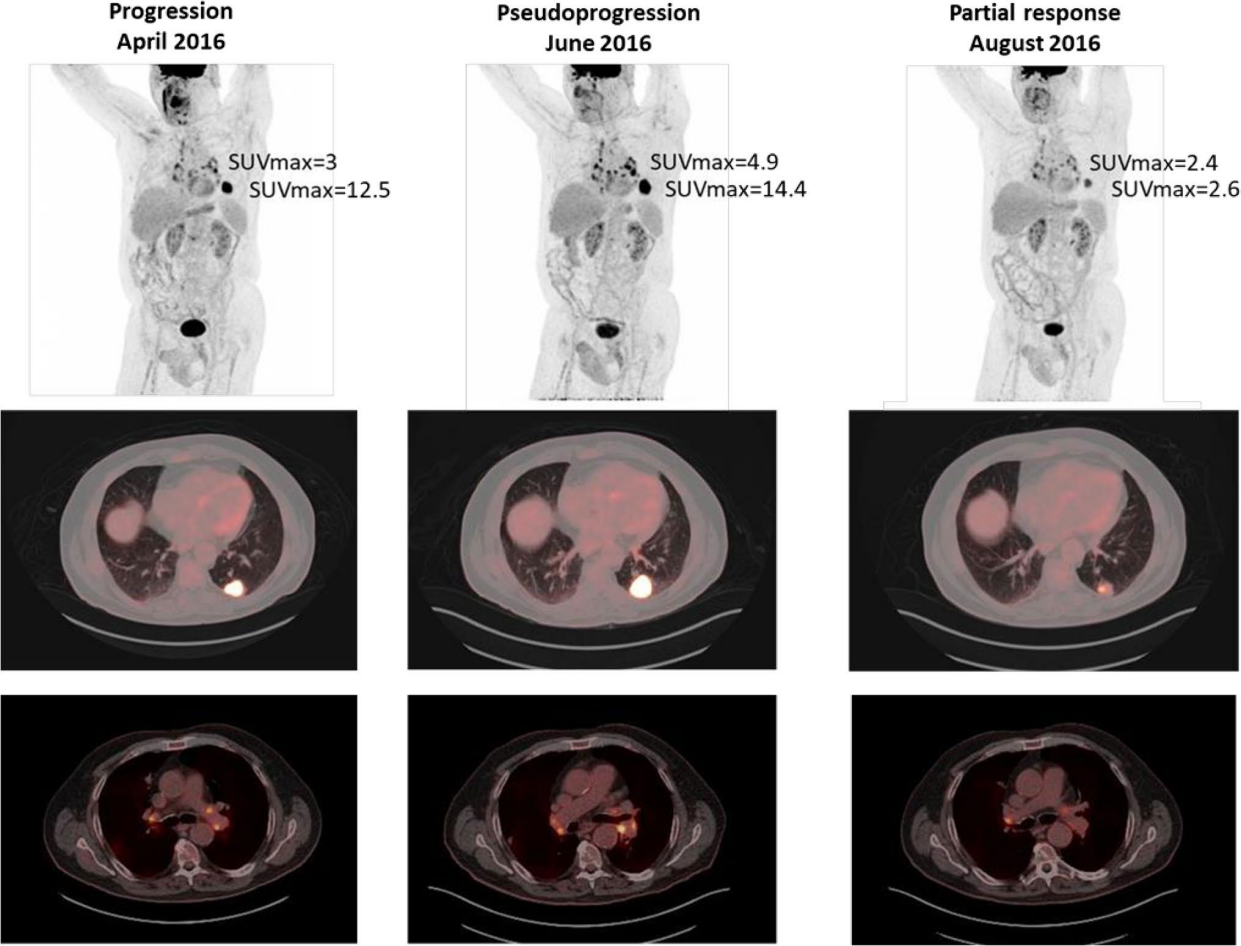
Pesudoprogression in a patient with lung adenocarcinoma. Patient diagnosed in January 2014 of well-differentiated lung adenocarcinoma T4N3M0 stage, and treated with cisplatin and pemetrexed. Progression resistant to a second-line chemotherapy was detected in April 2016. ^18^F-FDG PET/CT (a) identified a lesion located in LII (SUVmax of 12.5) and mediastinal and hilar lymph node uptake (SUVmax of 3). Nivolumab was started and an^18^F-FDG PET/CT study 2016 was performed for response assessment in June (b) revealing an increase in the metabolism and size of the lung lesion (SUVmax of 14.4) and in the number and metabolism of thoracic lymph nodes (SUVmax of 4.9). Because of clinical stability, immunotherapy was maintained and^18^F-FDG PET/CT performed in August 2016 (c) showed a metabolic reduction of lung lesion (SUVmax of 2.4) and lymph nodes (SUVmax of 2.6), consistent with partial response. Given the evolution, the ^18^F-FDG PET/CT(B) was interpreted as pseudoprogression
